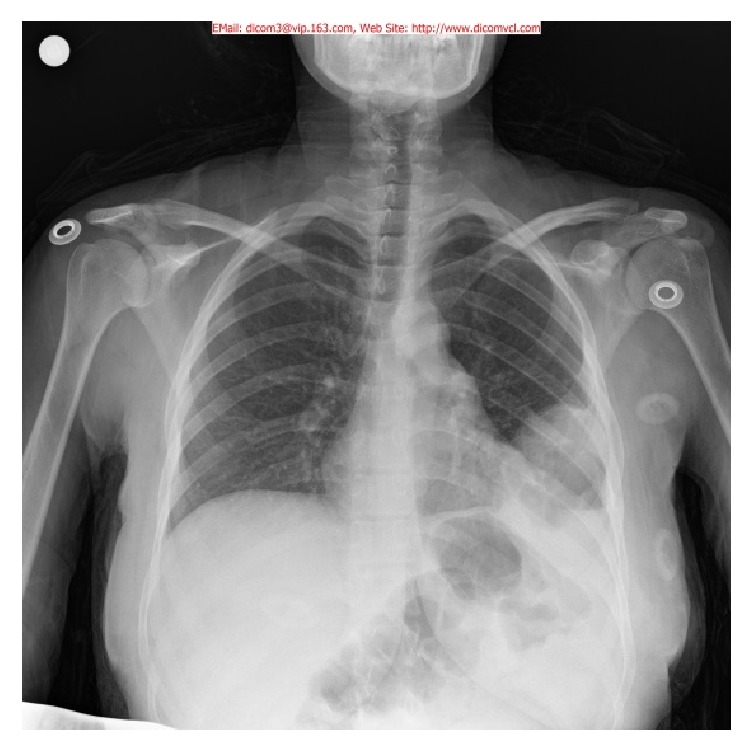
A herniated bowel loop in posteroanterior chest X-ray.
